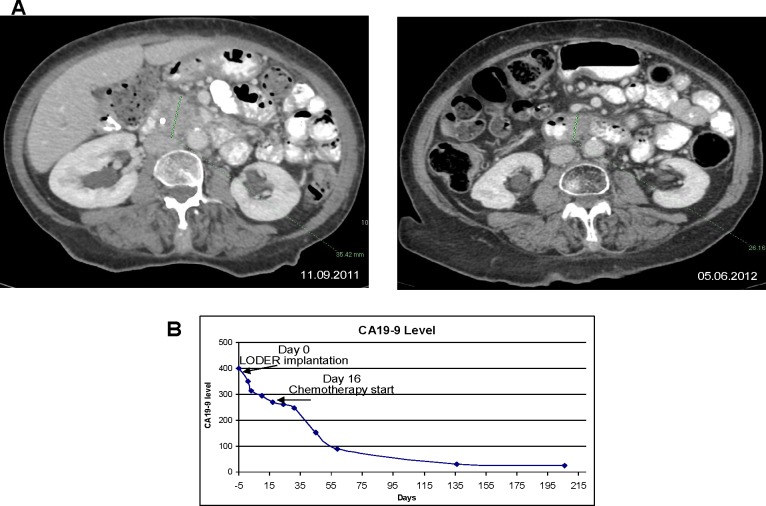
Anti-tumor effect of combination treatment with *siG12D-LODER^TM^* in locally advanced non-operable PAC in a patient A. left panel: a CT scan was performed prior to the implantation of *siG12D-LODER^TM^* using EUS; tumor measures 35.42mm in longest diameter; right panel: nine months later a significant tumor mass reduction is shown on a follow-up CT scan, tumor measures 26.16mm in the longest diameter. B. The level of CA19-9 in blood, showing 23% decrease immediately after *LODER^TM^* insertion, prior SOC treatment.
